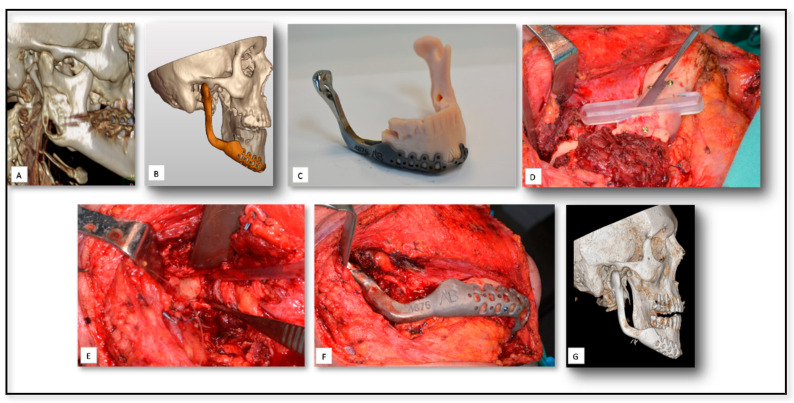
(A) A pre-operative 3D CT show the position and dimensions of the metastatic lesion. (B) 3D Planning including virtual removal of tumor and the virtual construction of the right temporomandibular joint (TMJ). (C) 3D printed stereolitic model and metal implant after virtual removal of Tumor in the right mandibular ramus. (D) The use of the cutting guide for accurate resection based on virtual cutting plan. (E) Removal of the tumor. (F): the placement of the printed TMJ implant. (G) Postoperative CT shows the accurate position of the TMJ implant.
